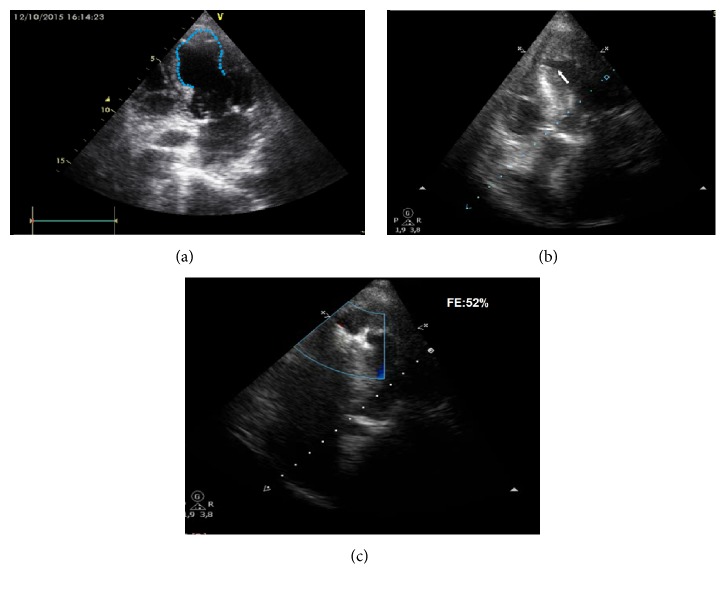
(a) Transthoracic echocardiogram (TTE) on admission, anterior and apical severe hypokinesia in both ventricles (apical ballooning pattern), and integrity of ventricular septum (dotted line and video); (b) TTE at the 4th day showing apical ventricular septal perforation (arrow) and a left ventricular ejection fraction of 41% (video); (c) TTE previous to discharge with the Amplatzer device and an improved left ejection fraction of 52%, with normal contractility in the right and left chambers (video).
